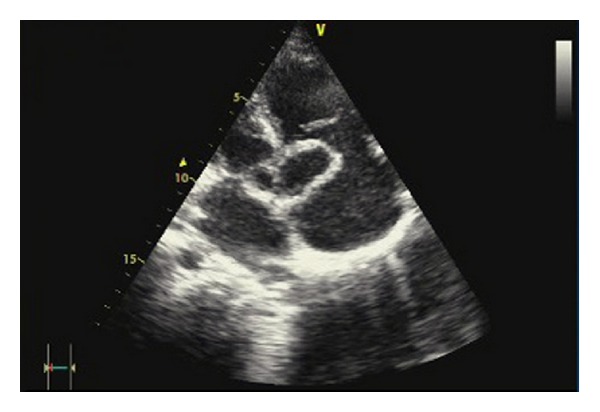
Sinus Valsalva aneurysm in apical five-chamber view.
